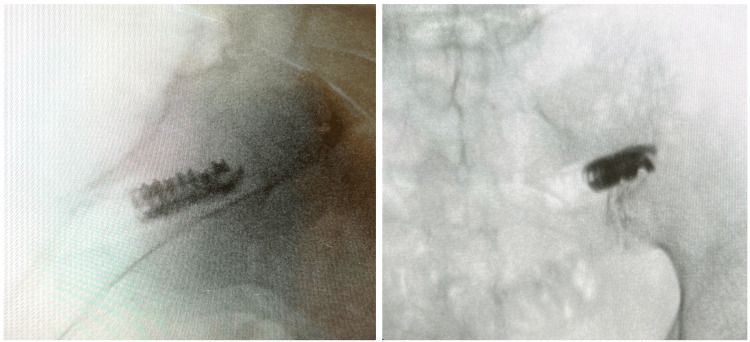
Intraoperative radiographs showing placement of the Patriot-SI™ in a 66-year-old female patient
